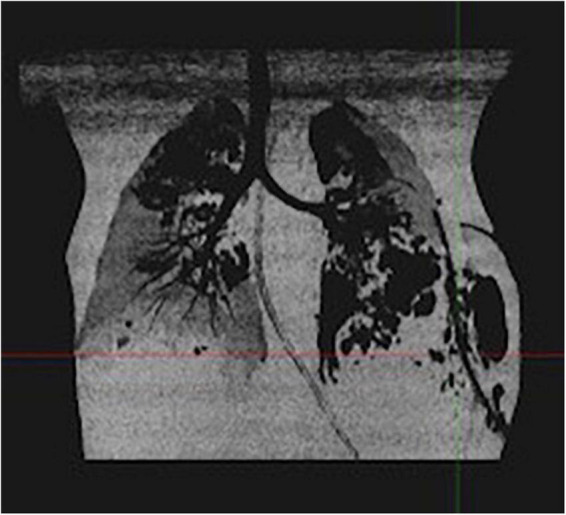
Thoracic CT scan of case 1: bilateral pneumatoceles and necrotizing pneumonia.
